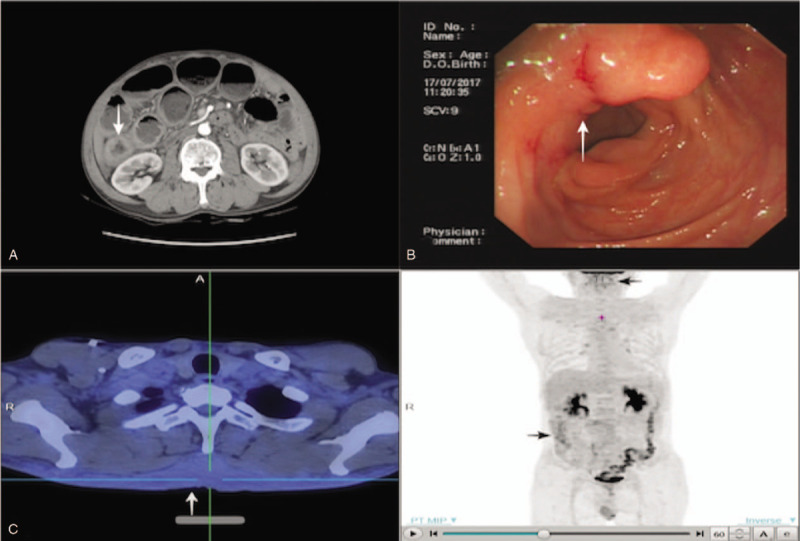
A: Abdominal CT revealed the lumen stenosis and the uneven thickening of the ascending colon and ileocecal region. B: Colonoscopy revealed that the surface of the ileocecal valves was rough and uneven, and the ascending colon presented as an annular stricture. C: FDG-PET/CT examination: This indicated a slightly abnormal 18F-FDG uptake at the adipose layer of the nape and bilateral scapular region, and the right hemicolon after chemotherapy.
